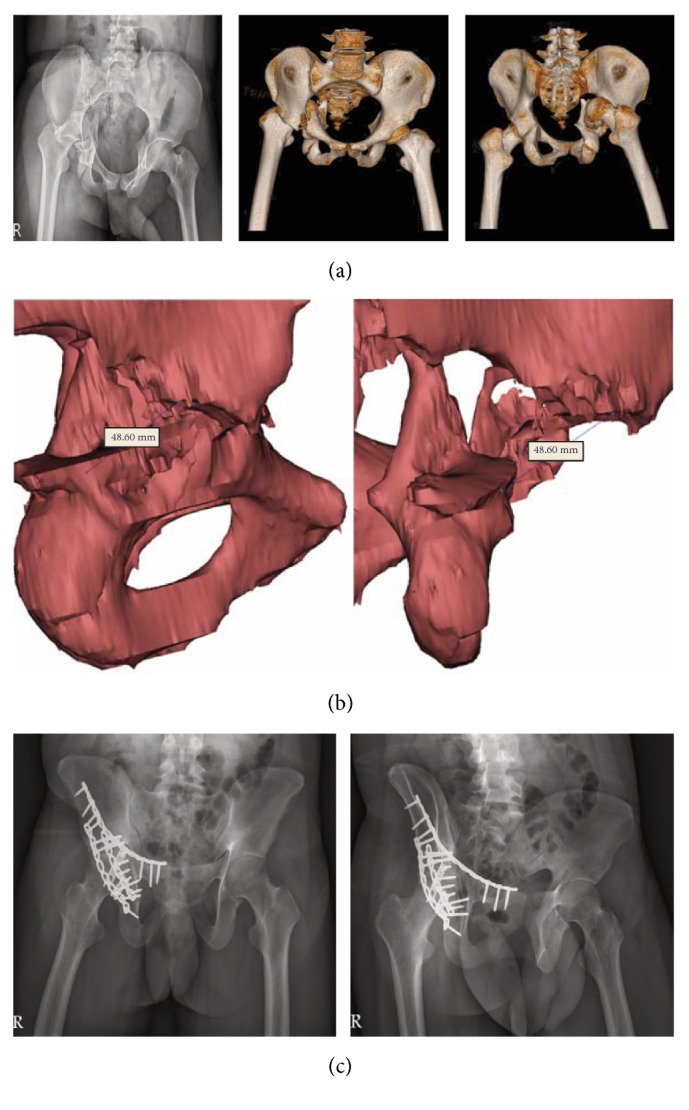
Images of posterior hip dislocation combined with acetabular fracture (T-shape + posterior wall) of the right acetabulum in a 22-year-old man: (a) preoperative pelvic plain film and CT scan film. (b) The posterior wall fragment length is about 48.60 mm as measured by the software. (c) The postoperative pelvic plain film reveals two spring plates, one posterior wall buttress plate, one posterior column plate, and one anterior column plate fixation.
